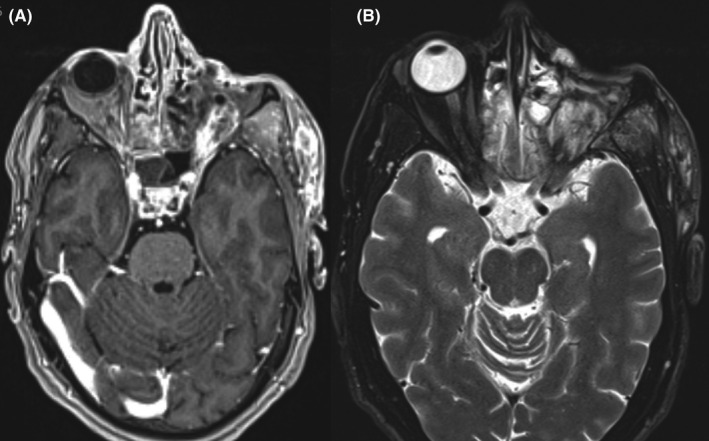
Brain magnetic resonance imaging (MRI) axial T1‐WI with gadolinium (A) and axial T2‐WI (B) showing the locoregional recurrence of the left orbital mass
